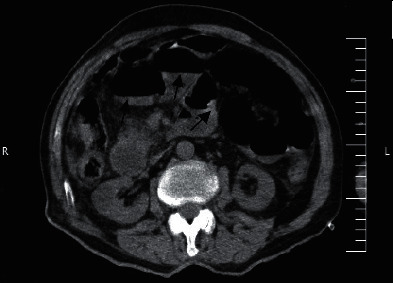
Male patient's abdominal CT (arrows: dilated small bowel loops).
